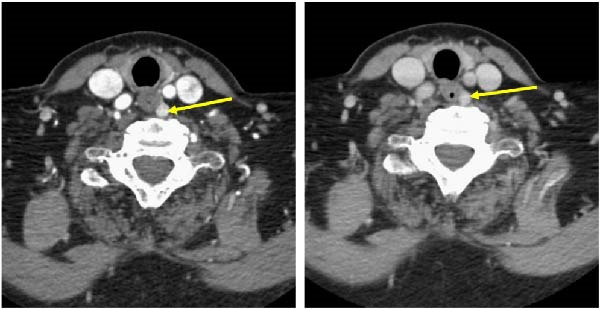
4D Neck CT with and without intravenous contrast. Identification of a hyperenhancing lesion in the neck at the thyroid level, separate from the thyroid gland and abutting the left posterior lateral margin of the esophagus concerning for a parathyroid adenoma.
